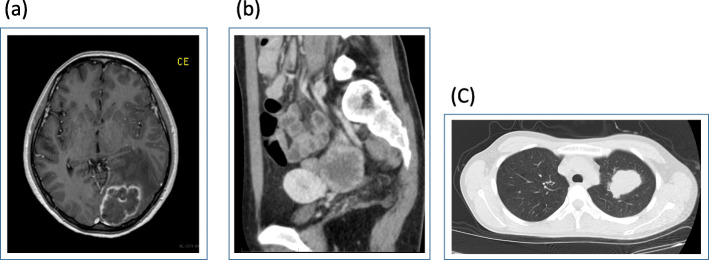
a Magnetic resonance imaging of the brain. Findings from contrast-enhanced T1-weighted magnetic resonance imaging of the brain. A tumor (4 cm in diameter) is seen causing midline shift in the left occipital lobe. The ridge of the tumor is enhanced. b Contrast-enhanced CT of the abdomen and pelvis. Contrast-enhanced CT of the abdomen and pelvis, showing a solid left ovarian tumor (5.5 cm in diameter) and enhanced with a ridge. c Chest CT Chest CT reveals metastatic choriocarcinoma in the left lung
